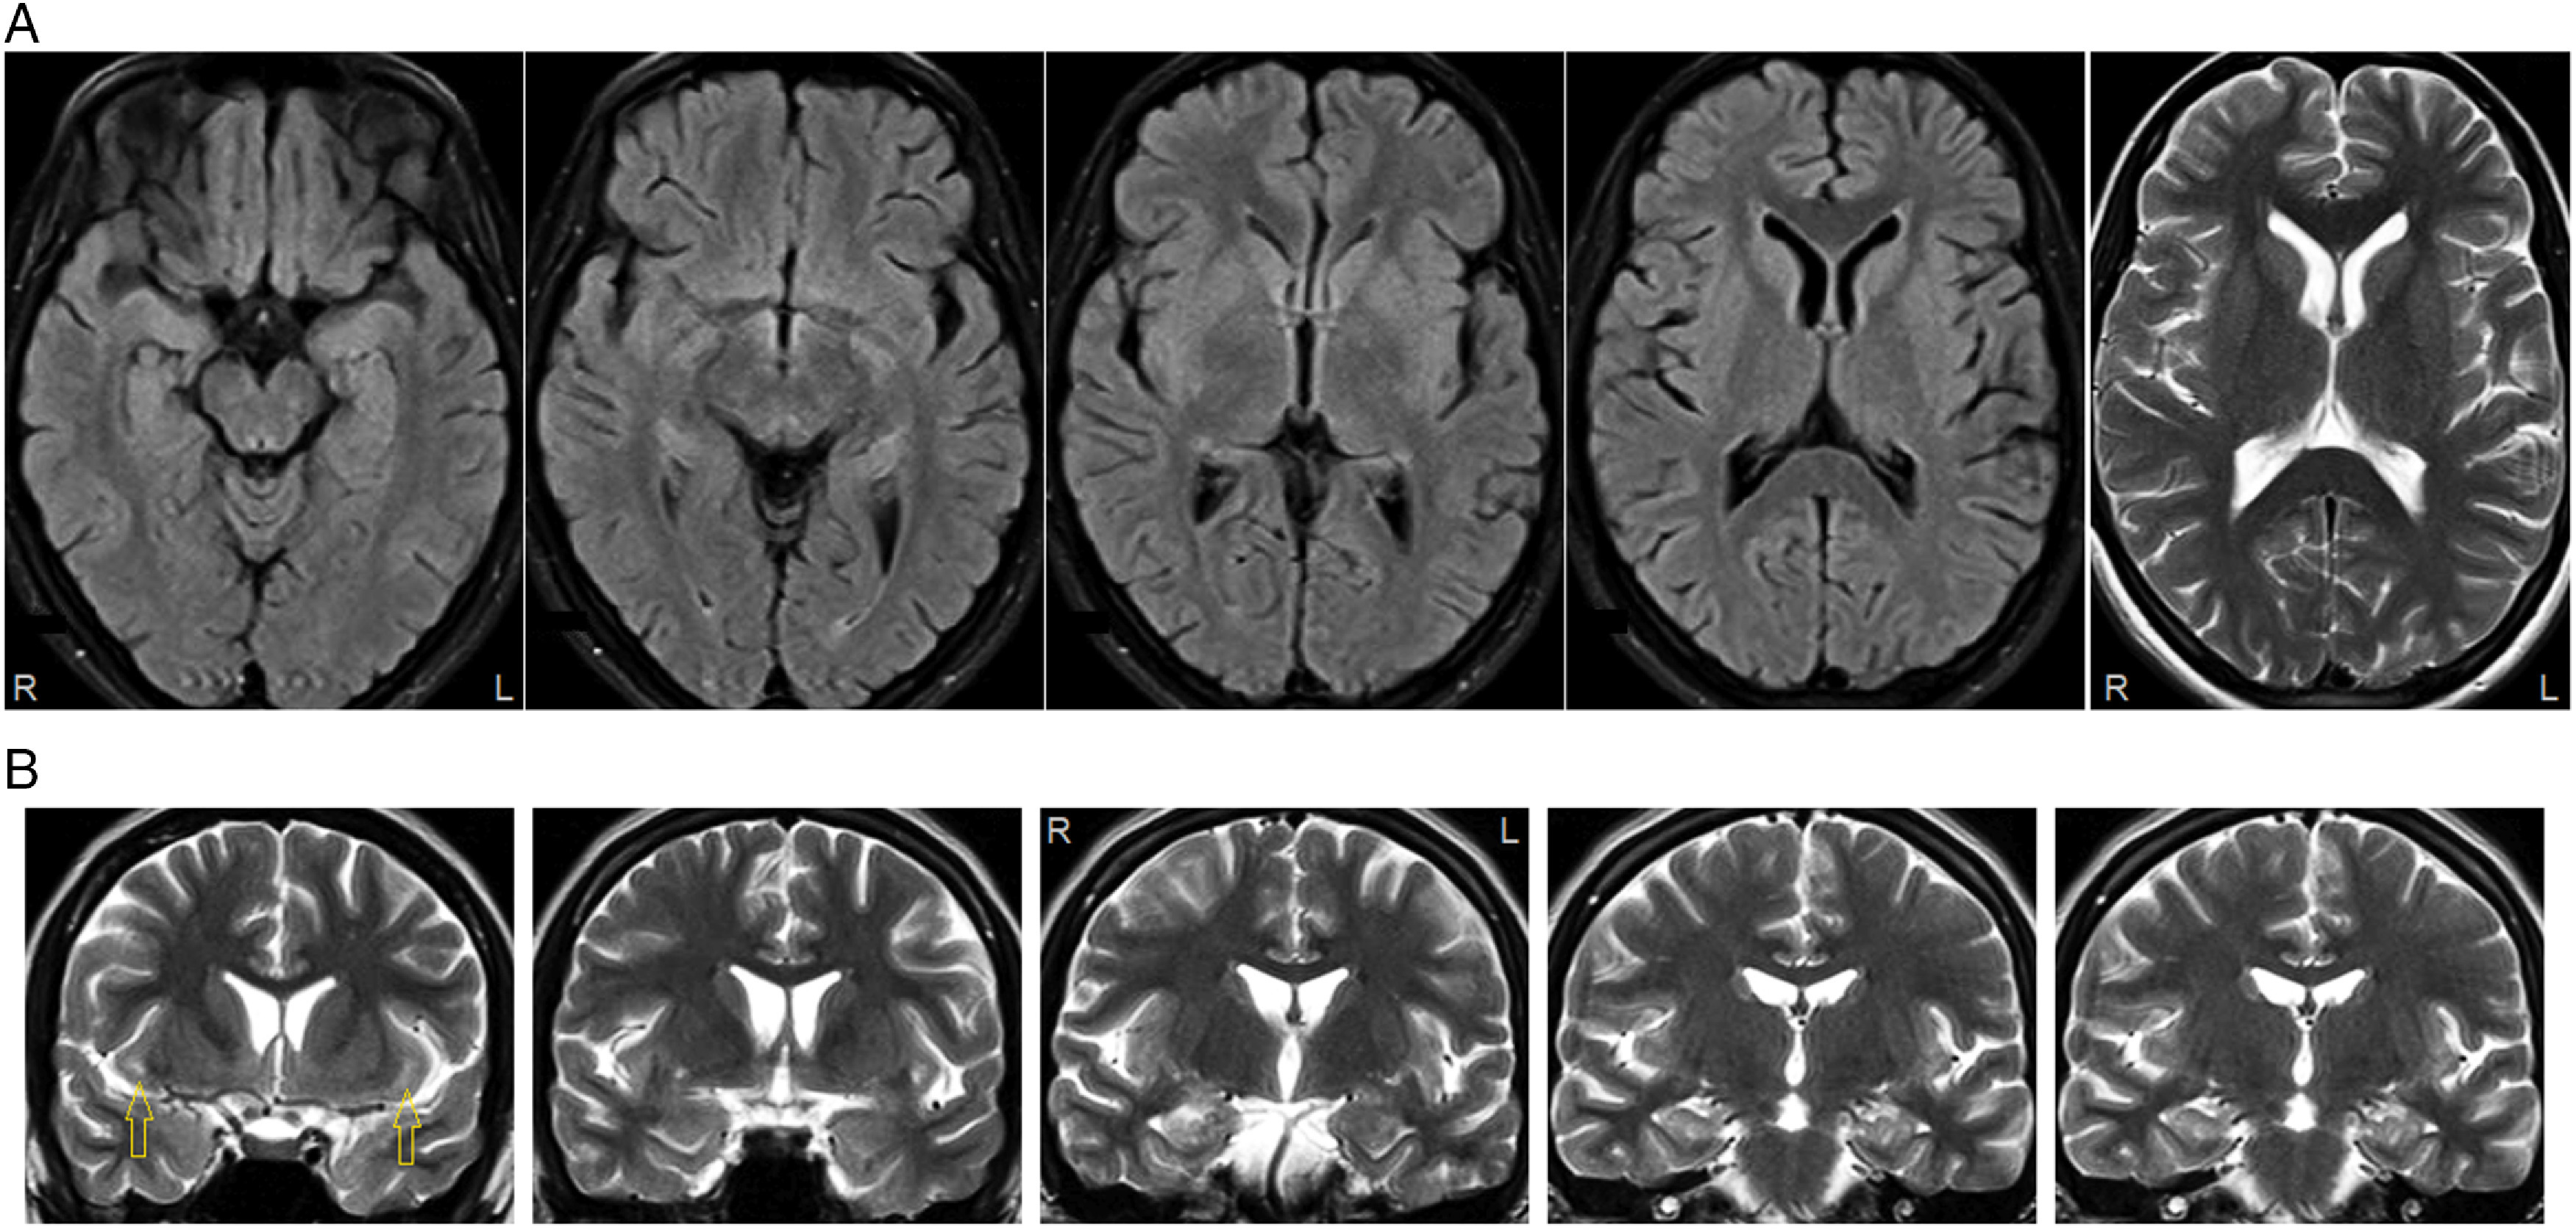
Follow-up brain MRI 1 month after scan shown in Figure 1. (A) Claustrum hyperintensities no longer evident on axial FLAIR or T2-weighted (far right panel) images. (B) Small areas of residual hyperintensity at anterior extent of claustrum and external/extreme capsules (left panel, arrows) on coronal T2-weighted images. Mesial temporal structures unremarkable.
